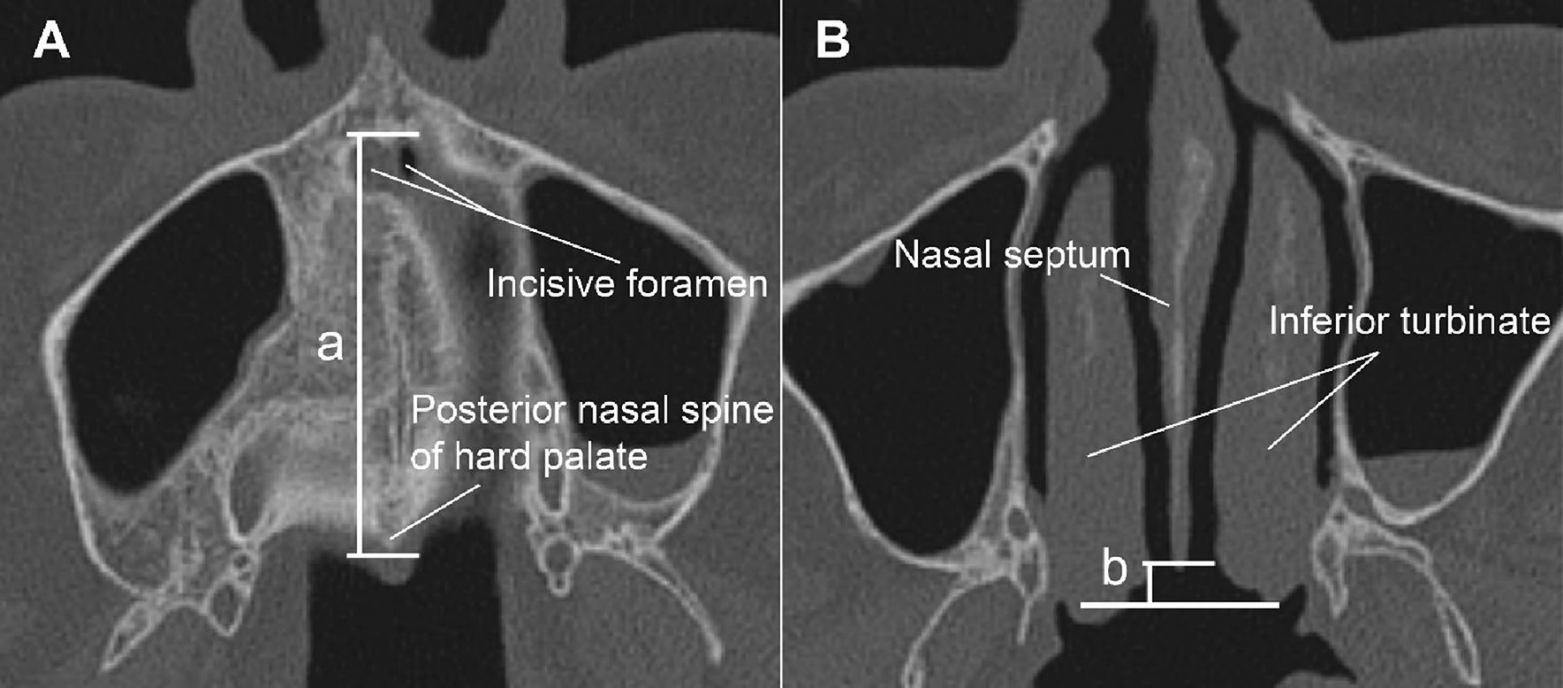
Measurement of vomer-maxilla fusion length (a) and vomer deformity (b) on computed tomography images of patients with a normal hard palate and vomer. (A) Vomer-maxilla fusion length is defined as the length from the incisive foramen to the posterior nasal spine of the hard palate. (B) Vomer deformity is defined when the posterior edge of the septum is ˃ 5 mm shorter than the shorter side of the posterior end of the inferior turbinate (b > 5 mm).
